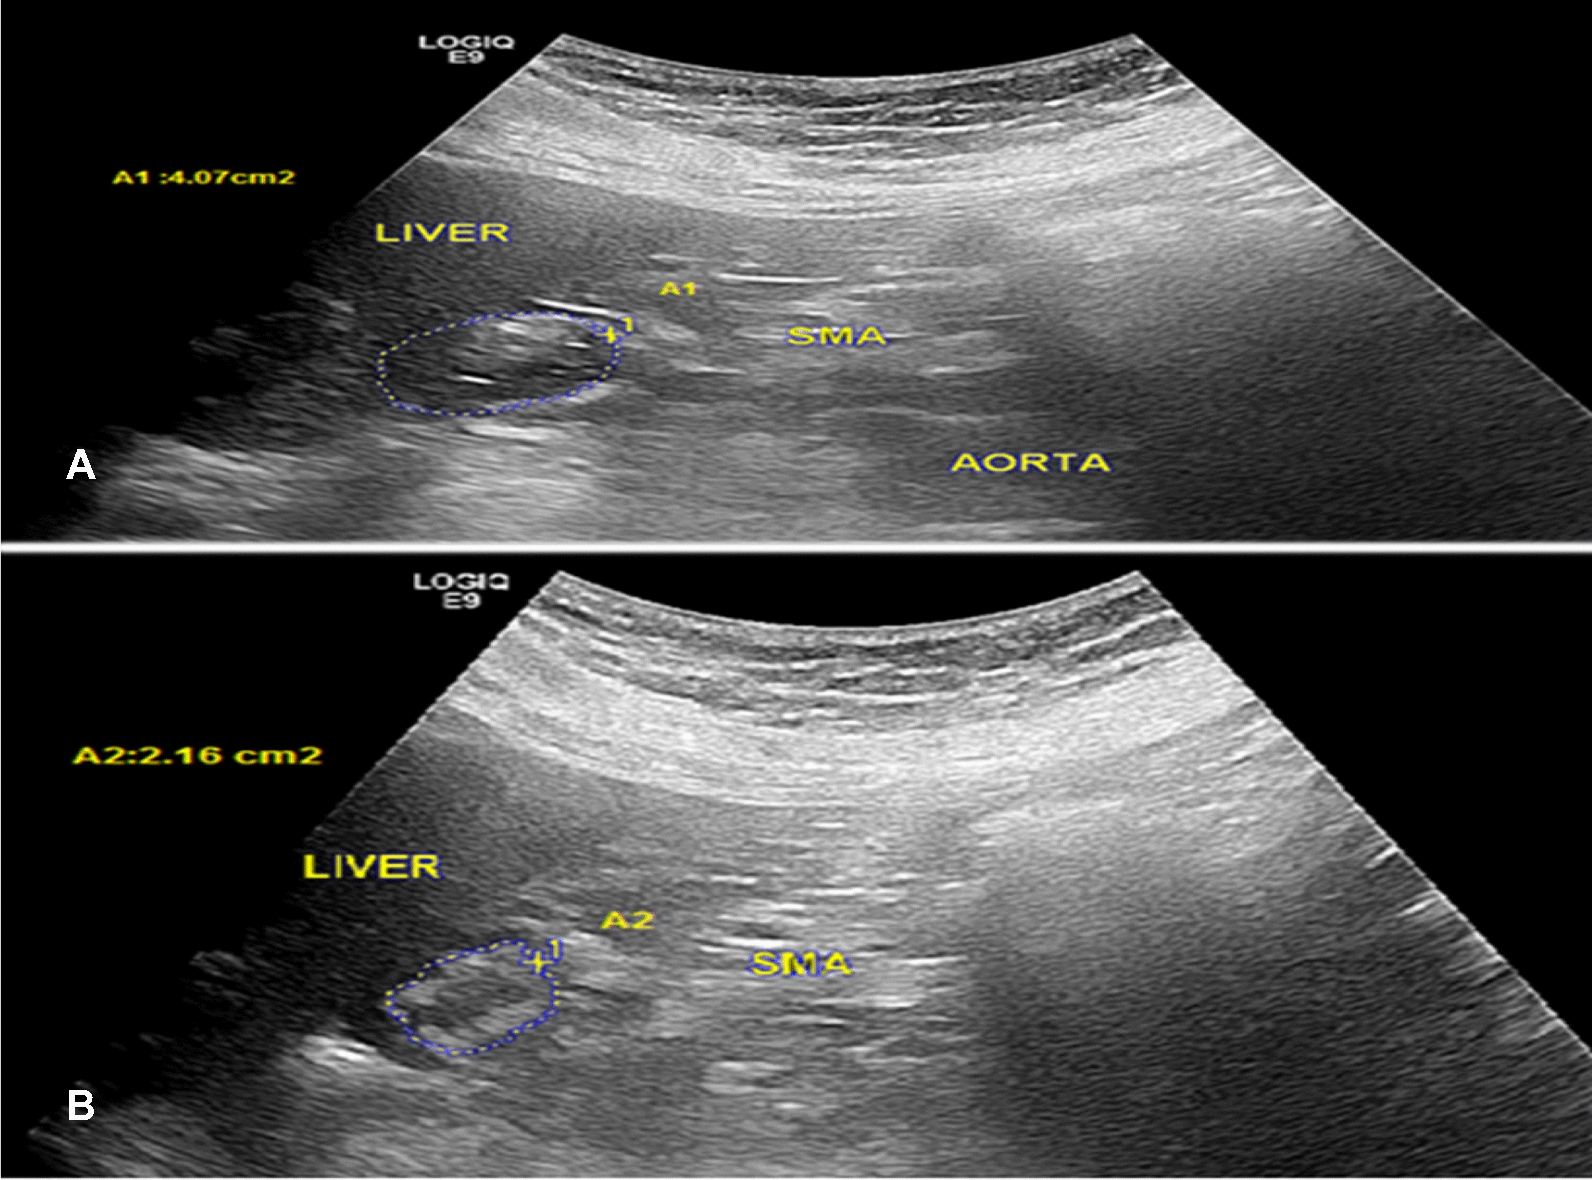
Vertically oriented ultrasonography demonstrates the relaxed phase at 1 min (A) and the contracted phase (B) at 3 min after ingestion of the meat soup. SMA: superior mesenteric artery, AO: aorta
